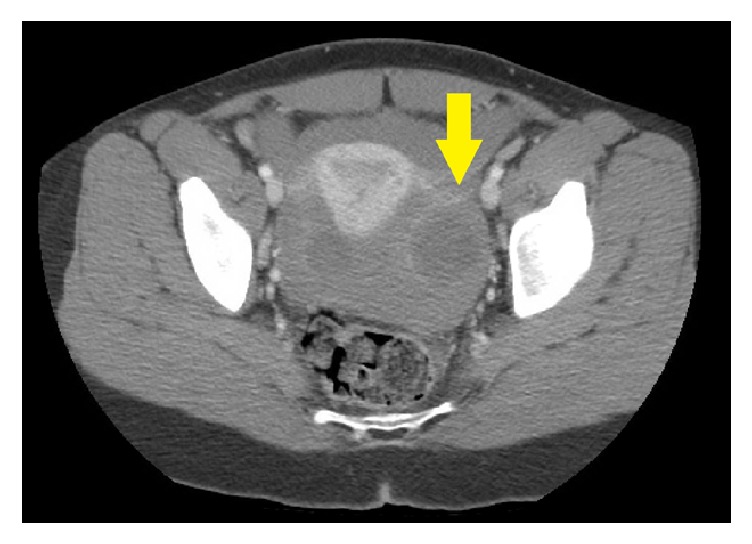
CT of the pelvis with contrast concentrated in fluid around left ovary (arrow).
